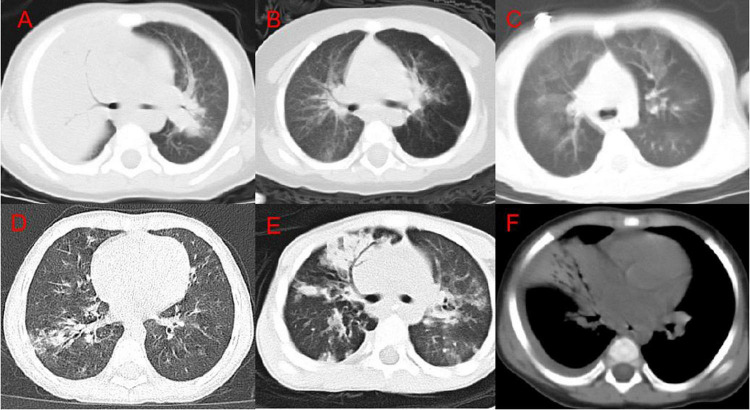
Chest CT imaging findings of adenovirus pneumonia. (A) Right upper lung consolidation with bronchial inflation sign; (B) left emphysema; (C) double lung mosaic sign; (D) right lower lung bronchial wall thickening and surrounding lobule inflammation; (E) right Middle lung bronchiectasis; (F) a small amount of pleural effusion on the right.
